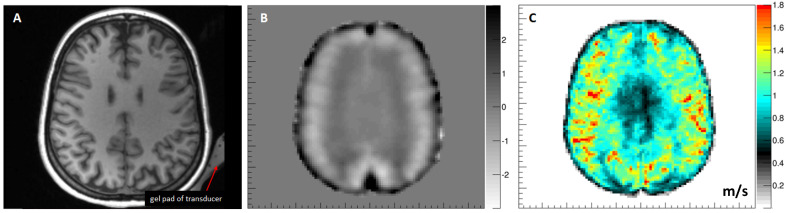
Three-dimensional MRE results in the brain. (A) Axial reformation of a T1-weighted 3D MPRAGE structural image of the brain. (B) Corresponding image of the Z-component of the curl showing the complex and intricate shear wave pattern within the brain. (C) The resulting image of the shear wave speed shows a very high level of symmetry within the brain parenchyma and low values within the lateral ventricles, as expected [m/s]. Data from University Hospital Heidelberg, Germany. The institutional ethical review board approved this prospective study. Written informed consent was obtained from the participants. The data were acquired in one of the investigators in preparation of a study approved by the Ethics Committee of Heidelberg University.
